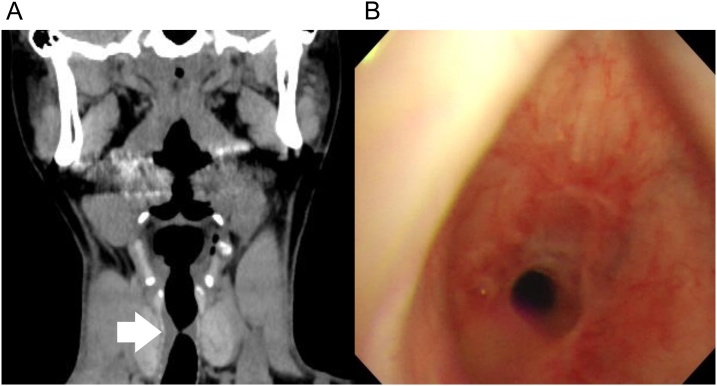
(A) Cervical/chest computed tomography scan (coronal view) taken at the onset, showing tracheal stenosis at the level of the 2nd to 4th rings (arrow). (B) Bronchoscopy image, showing a 75% constriction of the tracheal lumen due to cicatrization.
